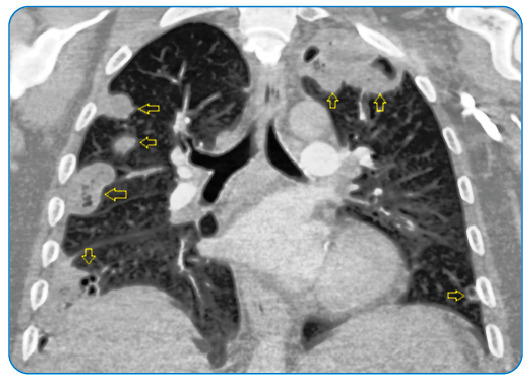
Coronal reformatted chest CT shows peripherally distributed nodules with or without cavitation (open yellow arrows).
